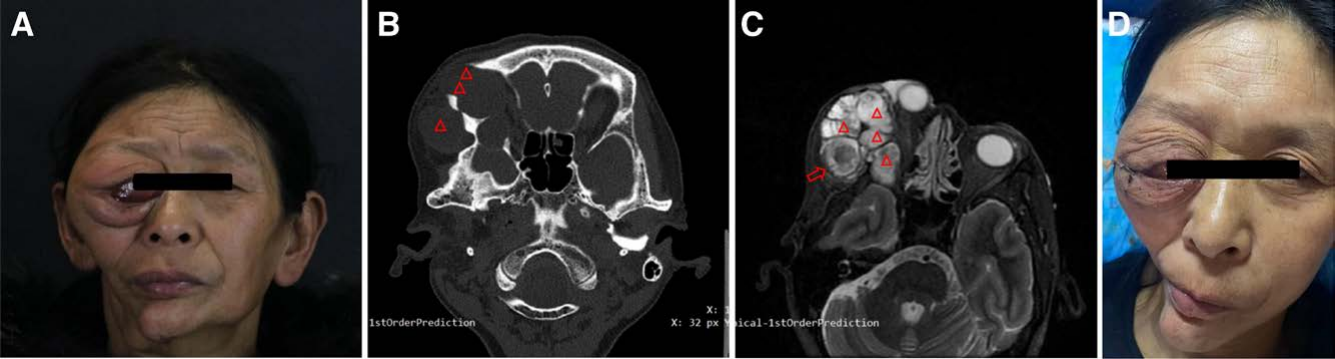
(A) The patient’s preoperative appearance. (B) Orbital CT (the tumor presents cystic changes, with the red triangle marking the tumor). (C) Orbital MRI (the red triangle marks the tumor, and the signal within the tumor cavity indicated by the red arrow is uneven). (D) The patient’s postoperative appearance. CT = computed tomography, MRI = magnetic resonance imaging.
